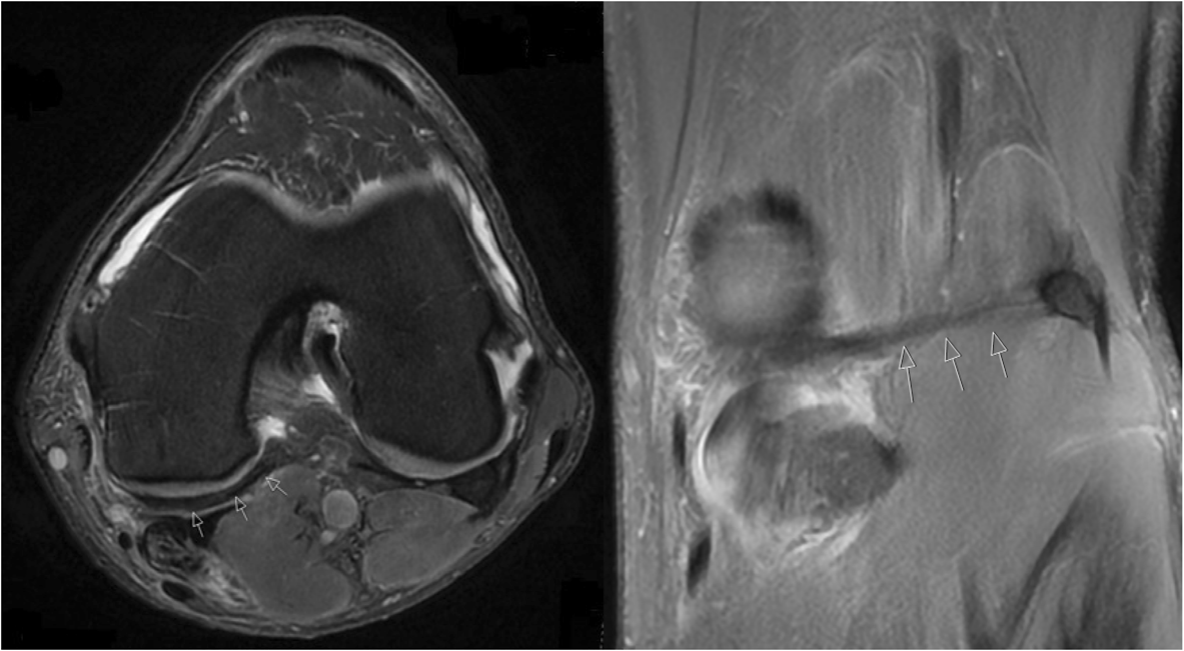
Axial and coronal magnetic resonance imaging images of the right knee, depicting the cord-like structure, originating from the fabella (*arrowed*)
